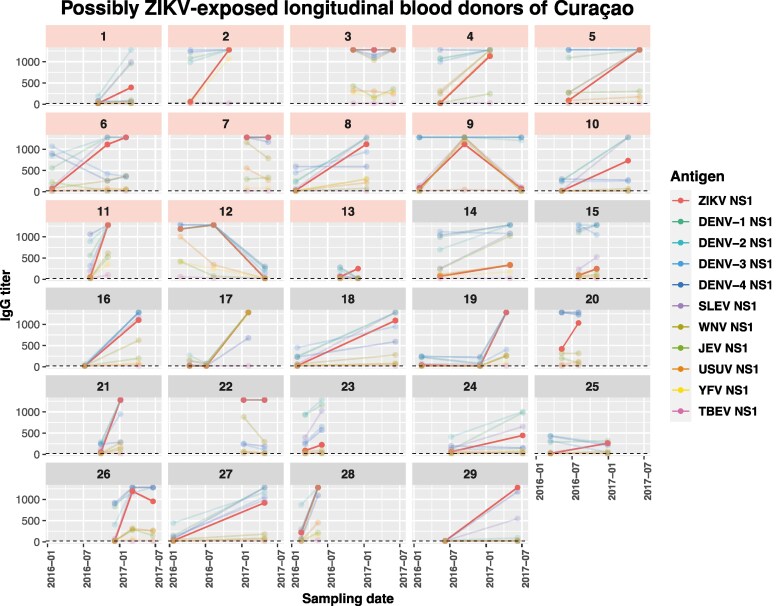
Flavivirus nonstructural protein 1 immunoglobulin G over time of longitudinal blood donors (2 or 3 timepoints) that possibly reflect Zika virus (ZIKV) exposure (N = 29). Donors 1–13 are ZIKV confirmed based on the virus neutralization test (pink panels). Abbreviations: DENV, dengue virus; IgG, immunoglobulin G; JEV, Japanese encephalitis virus; NS1, nonstructural protein 1; SLEV, Saint Louis encephalitis virus; TBEV, tick-borne encephalitis virus; USUV, Usutu virus; WNV, West Nile virus; YFV, yellow fever virus; ZIKV, Zika virus.
